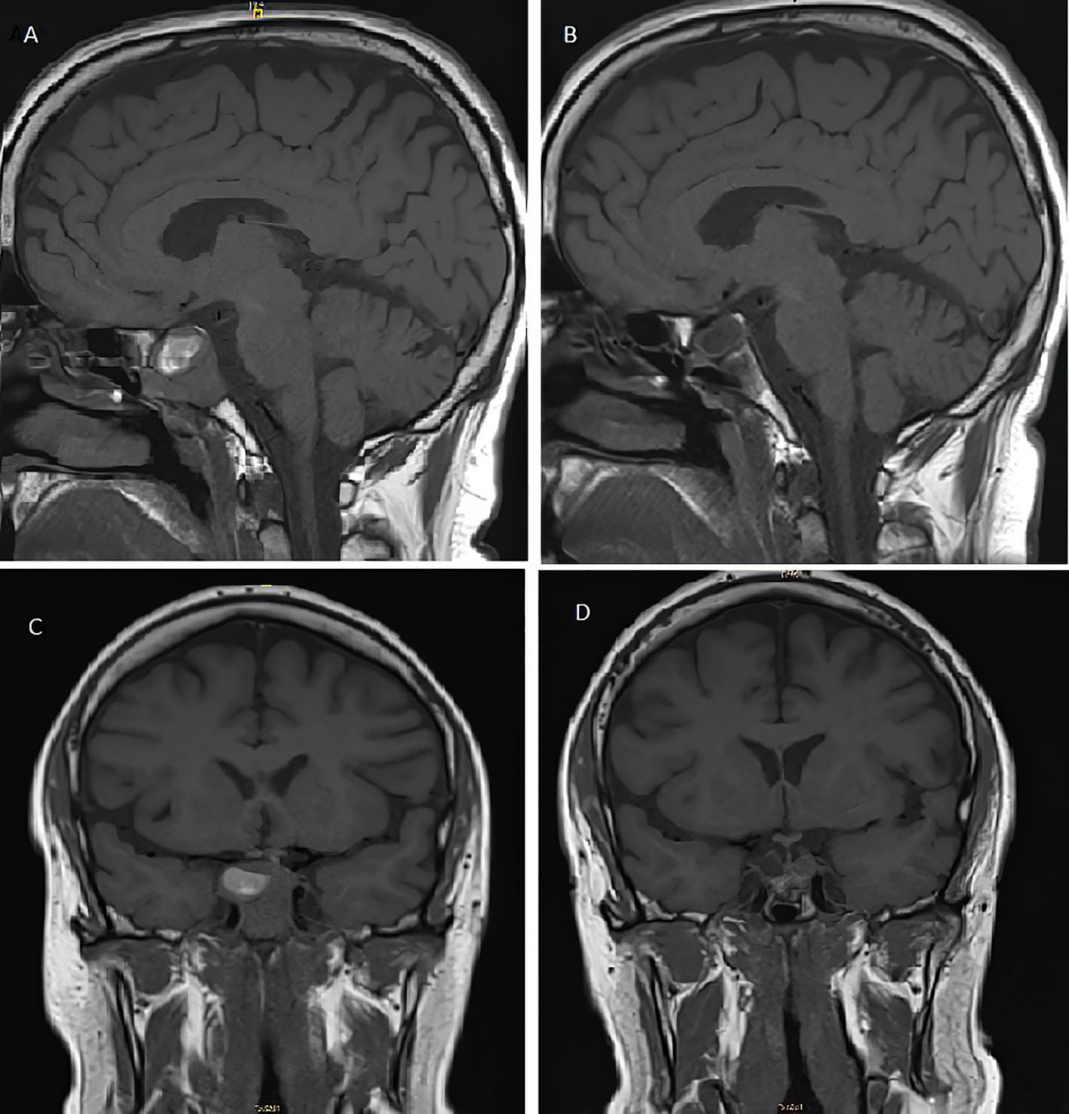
T1-weighted pituitary magnetic resonance imaging before (A, C) and after (B, D) treatment with capecitabine and temozolomide. (A) Pre-CAPTEM sagittal image shows an increase in the size of the seal tumor (26 mm) with a subacute bleeding component. (B) Post-CAPTEM sagittal image shows a decrease of 65% with a total size of 9 mm. (C) Pre-CAPTEM pituitary coronal image shows invasion of clivus and protrusion into the sphenoid sinus. (D) Post-CAPTEM coronal image shows a decrease in size of the lesion, more prominent at the right level.
